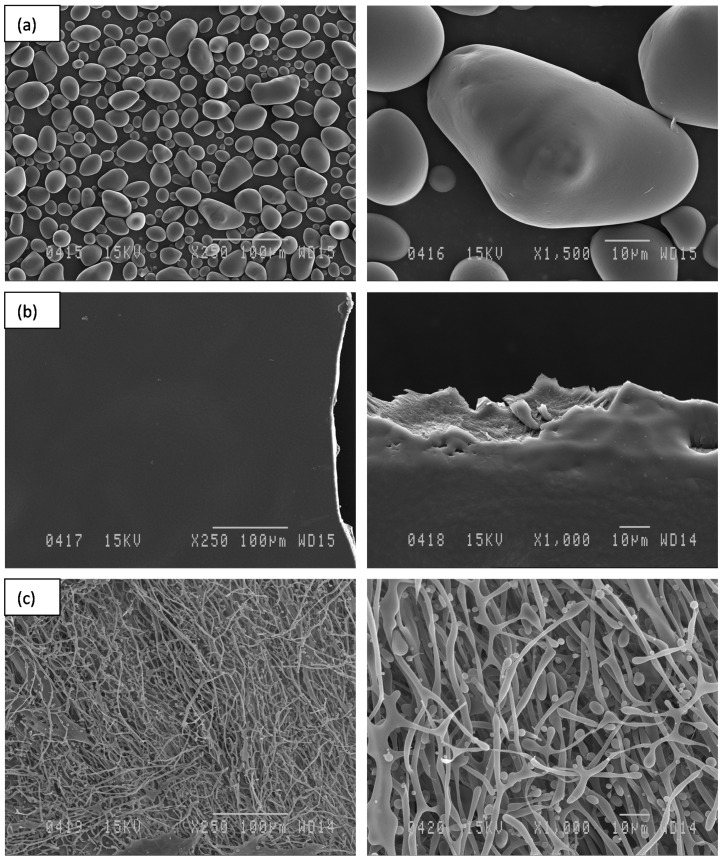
SEM images of potato starch granules treated with ultrasonication under dry conditions (a); under wet conditions and dried in a vacuum oven at 40 °C (b); under wet conditions and lyophilized (c).
